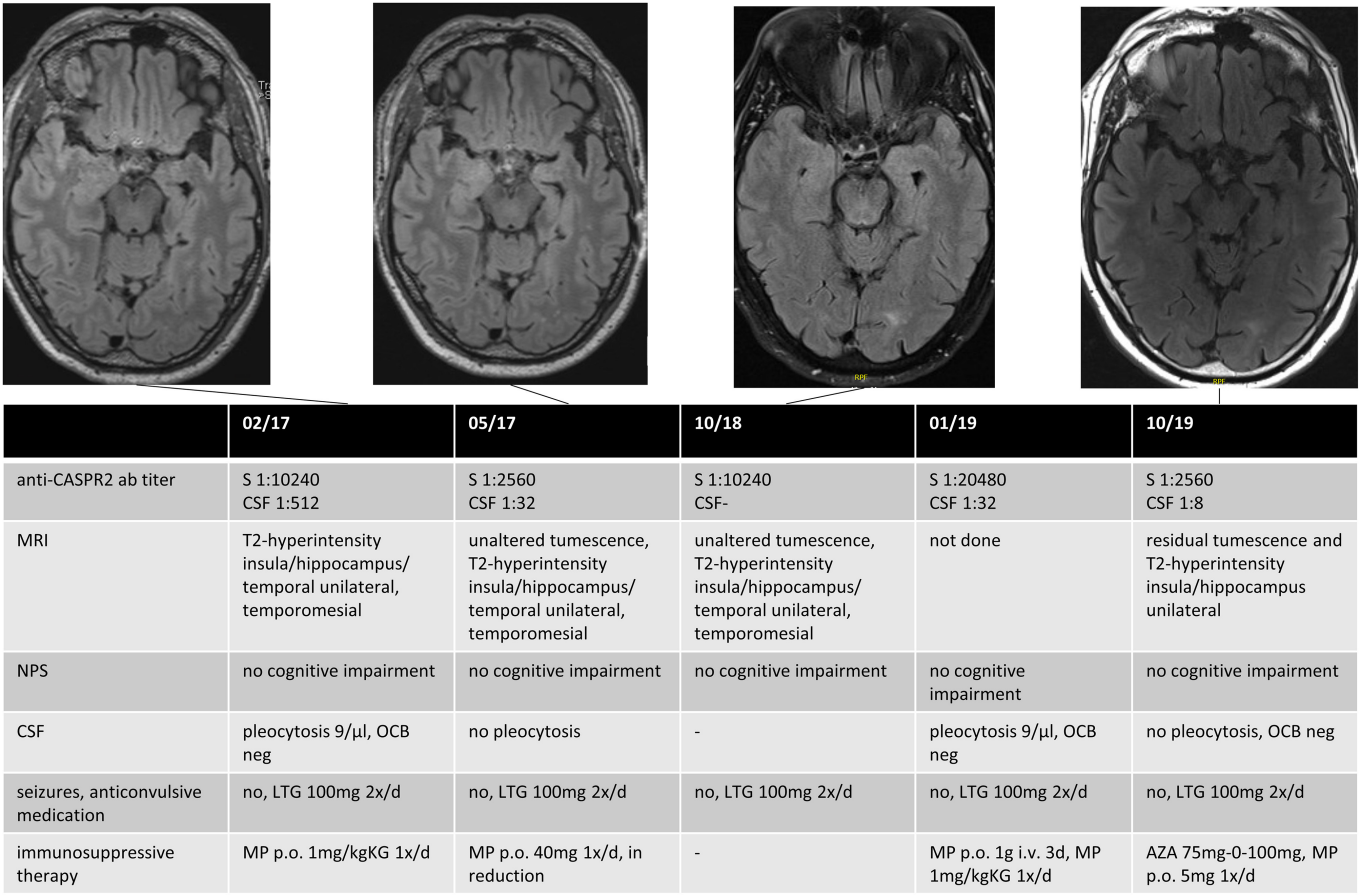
Data for an anti-CASPR2 AB positive patient. The patient had a first epileptic seizure (GMS) 3 months before the diagnosis and recurrent focal seizures until he received monotherapy with Lamotrigin, after which he became seizure-free. The first MRI showed unilateral swelling and T2-hyperintensity in the insula, the hippocampus, temporal mesial, and in the temporal lobe, compatible with possible AE, although not fulfilling the Graus criteria in the absence of neurocognitive or neuropsychiatric deficits. Immunotherapy was initialized after positive testing for anti-CASPR2 AB in serum and CSF. The patient had no clinical signs of AE until last FU; immunotherapy was reinitialized 2 years after diagnosis because of an increased titer of anti-CASPR2 AB in routine clinical FU and a recurrent pleocytosis in CSF. Anti-CASPR2 contactin-associated protein-like 2, GMS generalized motor seizure, MRI magnet resonance imaging, AE autoimmune encephalitis, CSF cerebrospinal fluid, FU follow-up, S Serum, NPS neuropsychological testing, OCB oligoclonal bands, LTG Lamotrigin, MP Methylprednisolon, AZA Azathioprin.
